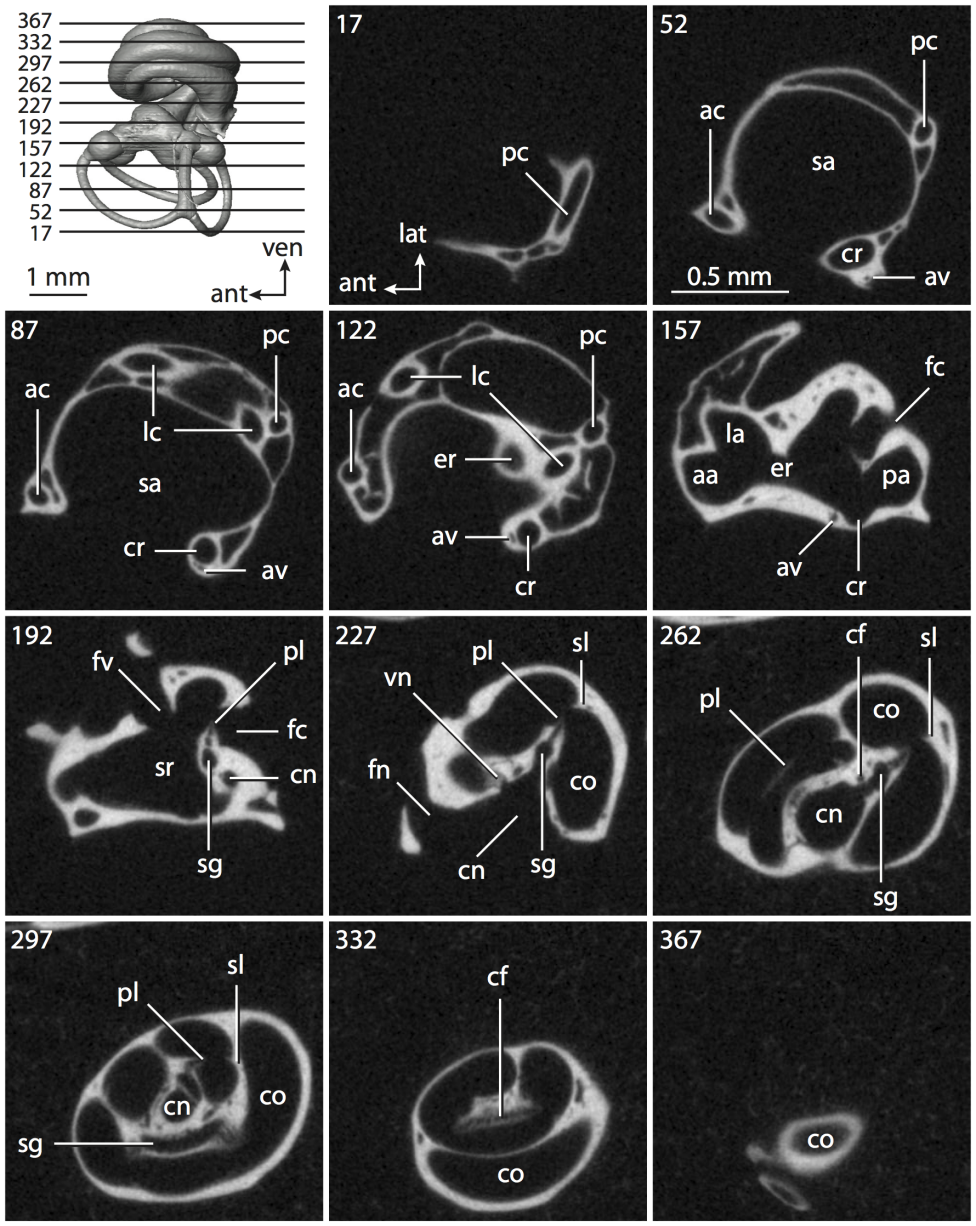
CT slices through ear region of*Tadarida brasiliensis*. Abbreviations listed at the end of the Materials and Methods section.
